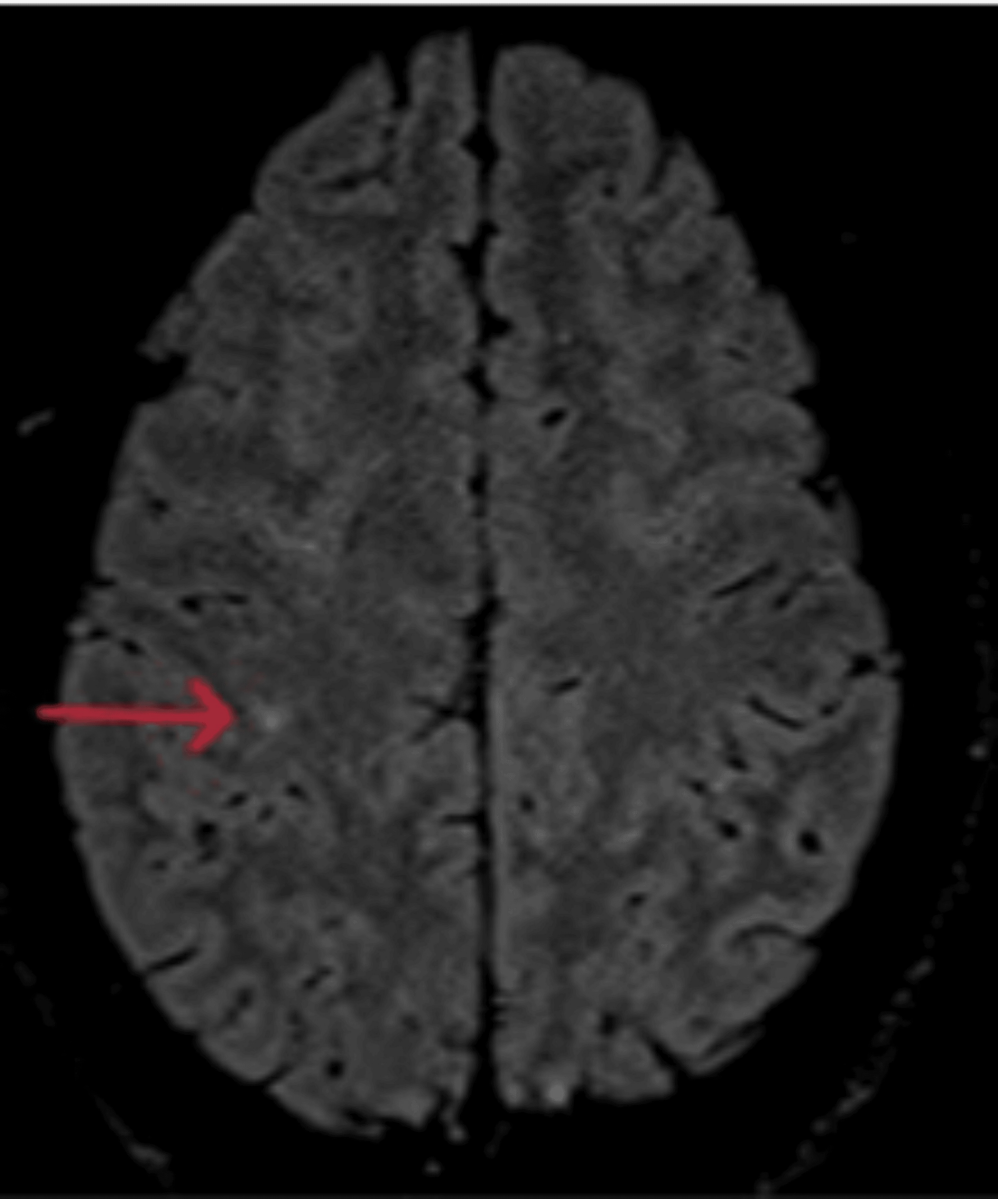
Post-contrast FLAIR showing leptomeningeal enhancement in bilateral high frontoparietal sulci. FLAIR: Fluid-attenuated inversion recovery
